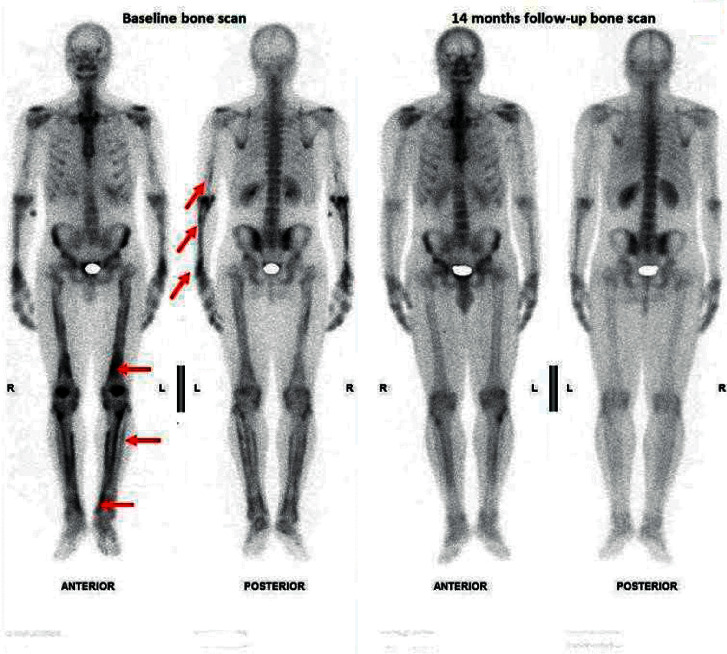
(a) Bone scan showing increased radiotracer uptake (arrow) along the tubular bones of the upper and lower extremities consisting with periostosis. (b) The whole-body scan performed 14 months later showing the improvement of the scintigraphic abnormalities.
